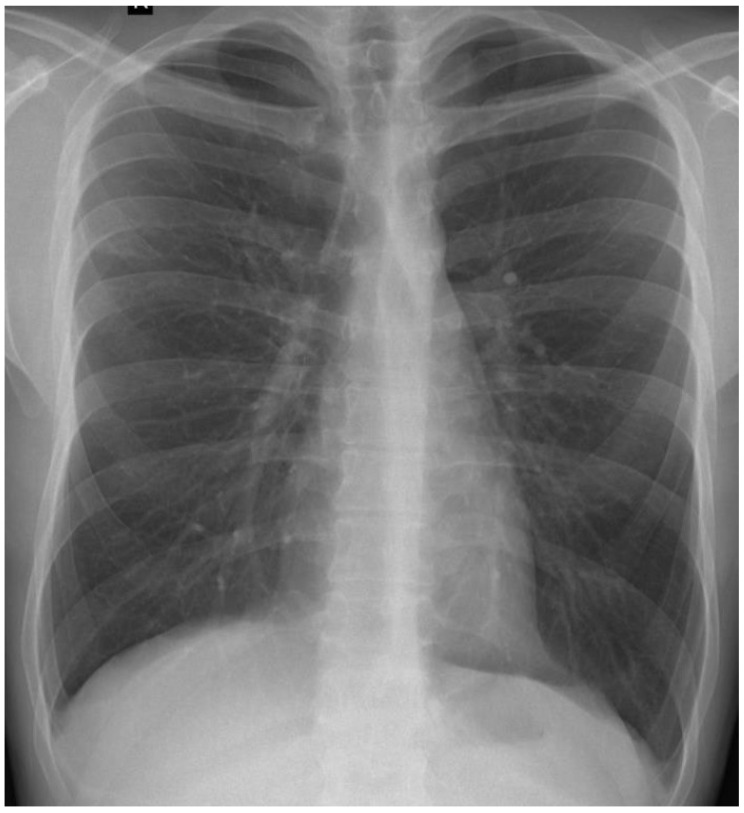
Chest X-ray at T6 shows complete resolution of right suprahilar opacity and no evidence of residual sequelae.
